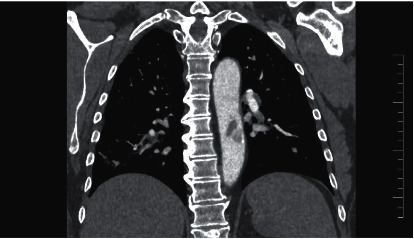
Fluttering aortic thrombosis on CT angiography (case no. 2).
